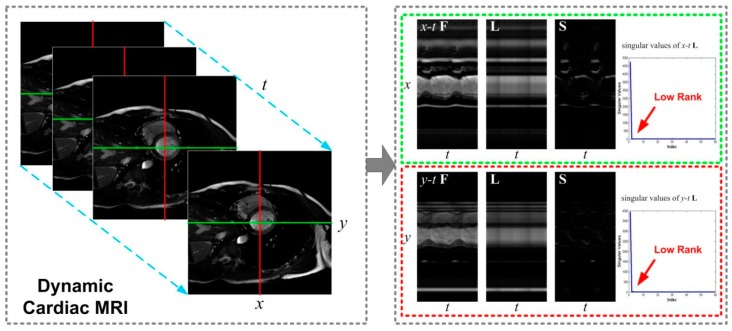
The low-rank plus sparse (L + S) decomposition of a fully sampled 2D axial cardiac MRI dataset. RPCA decomposes the x-t (or *y*-*t*) temporal profiles (i.e., Casorati matrix) F into a low-rank component L and a sparse component S. The singular values of both *x*-*t* and *y*-*t*
